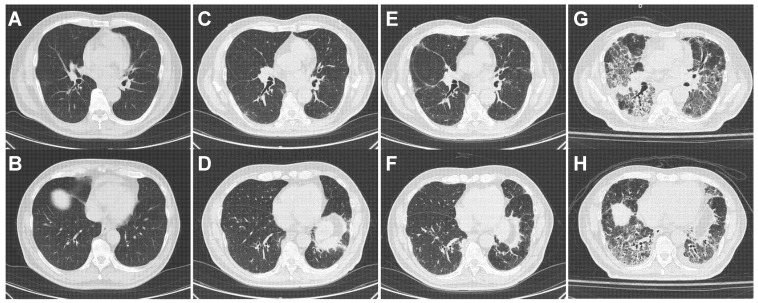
Case 2, a 68-year-old man. CT images. (A,B) Before treatment. (C,D) After four cycles of carboplatin, pemetrexed, and pembrolizumab. (E,F) After an additional four cycles of pembrolizumab. (G,H) After an additional 3 months with best supportive care.
